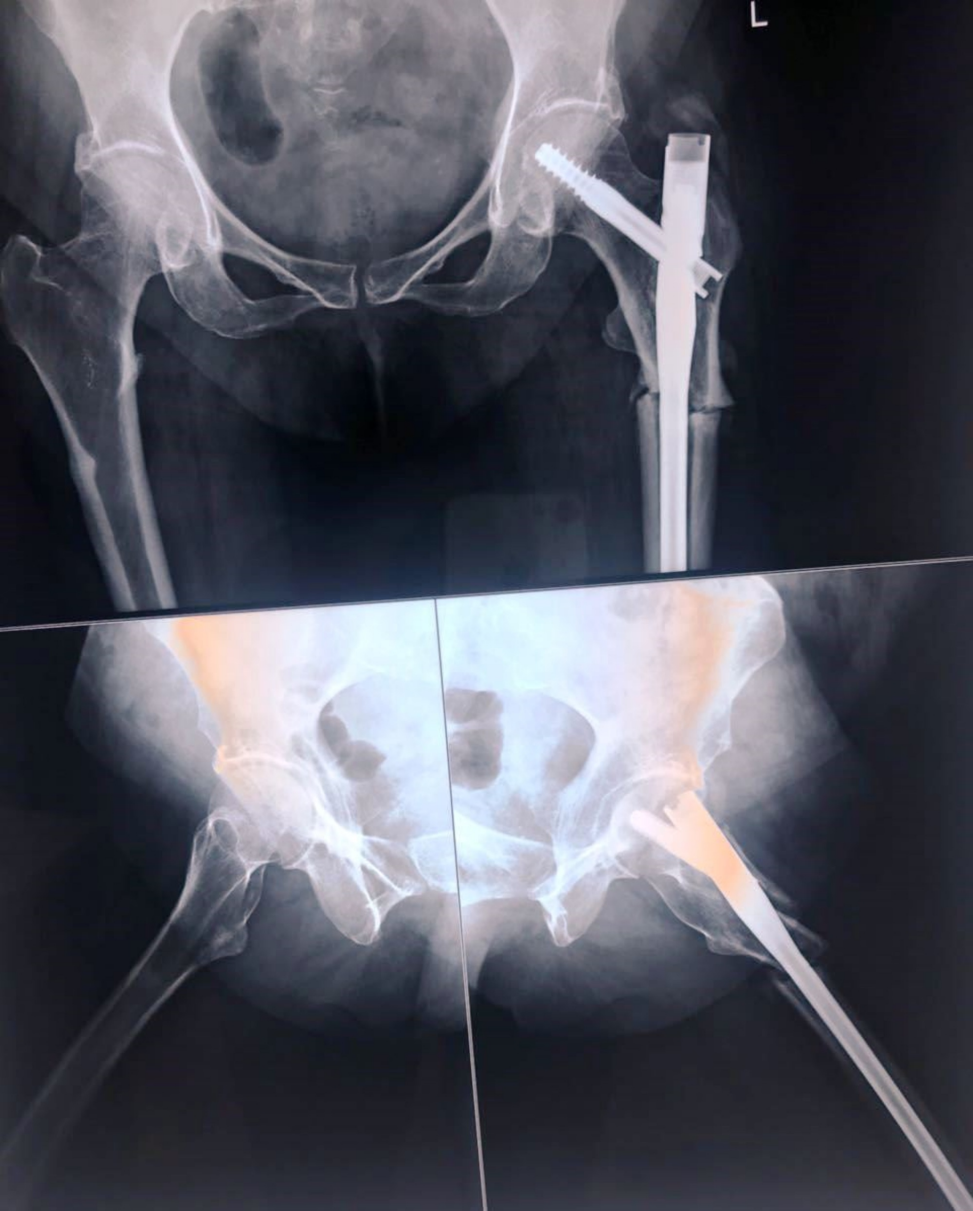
Immediate postoperative X-ray showing the intramedullary nail in situ.
